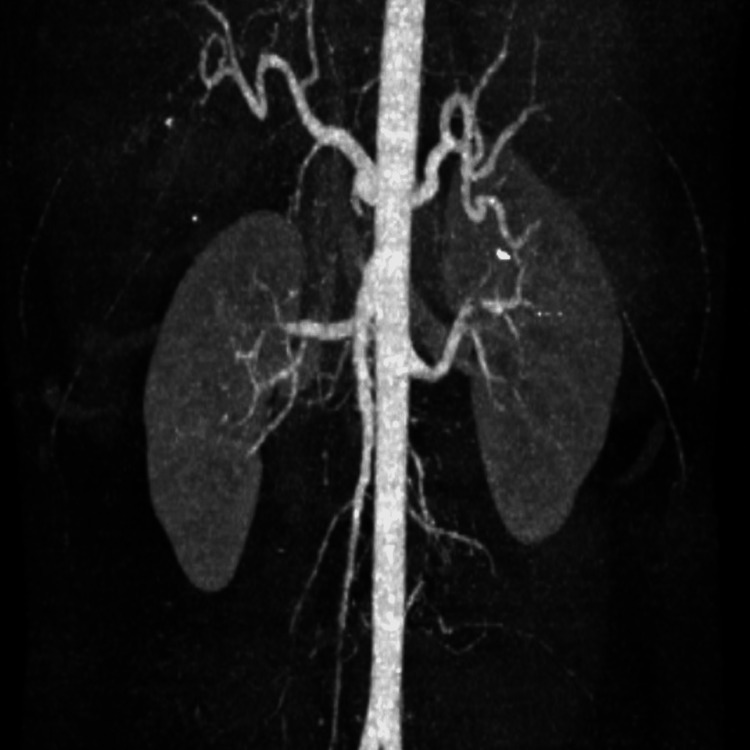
CT angiogram of the abdomen showing absence of a splenic artery pseudoaneurysm
